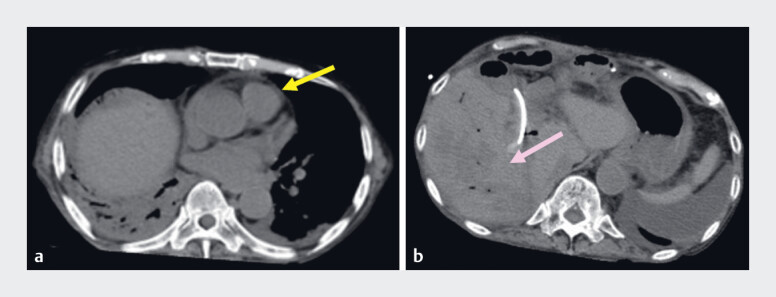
a Computed tomography done 2 h after resuscitation showed:
